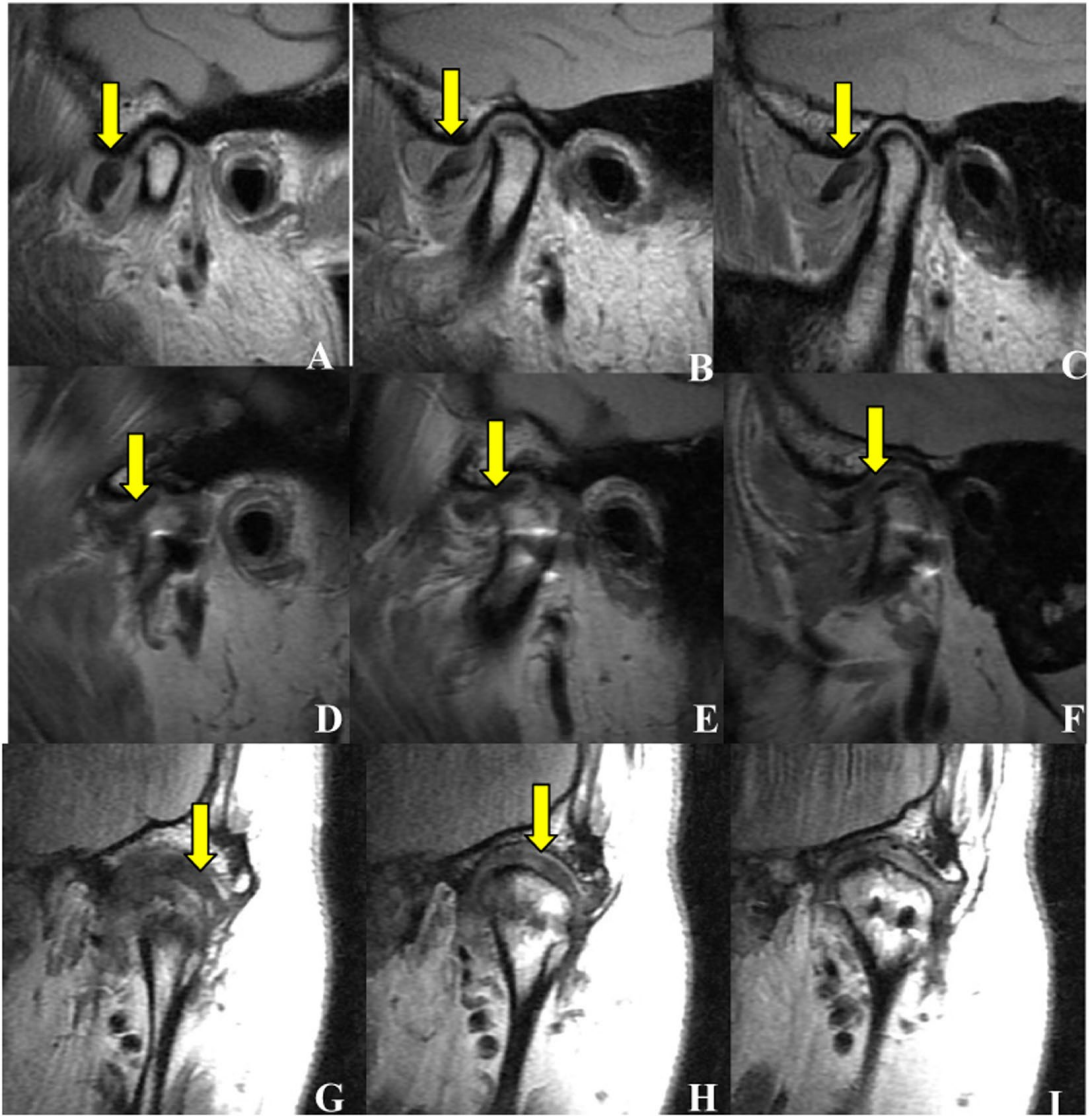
Disc position visualized by MRI before and after surgery – example of B classification. Internal (A), middle (B) and lateral (C) layers of the long axis of the condylar process shown before surgery, in the sagittal plane. The images demonstrate that the disc is anteriorly displaced in the closed-mouth position (yellow arrow). Internal (D) and middle (E) layers of the long axis of the condylar process after surgery, in the sagittal plane. The images demonstrate that the displaced disc is repositioned in the normal position (yellow arrow), while in the lateral (F) layer the displaced disc is not repositioned (yellow arrow). Anterior (G) and middle (H) layers of the long axis of the condylar process, after surgery, in the coronal plane. The images demonstrate that the displaced disc is repositioned in the normal position (yellow arrow), while in the posterior (I) layer the displaced disc is not found. As the disc is re-set in two layers (internal and middle layers in the sagittal plane, and anterior and middle layers in coronal plane) the case was classified B.
